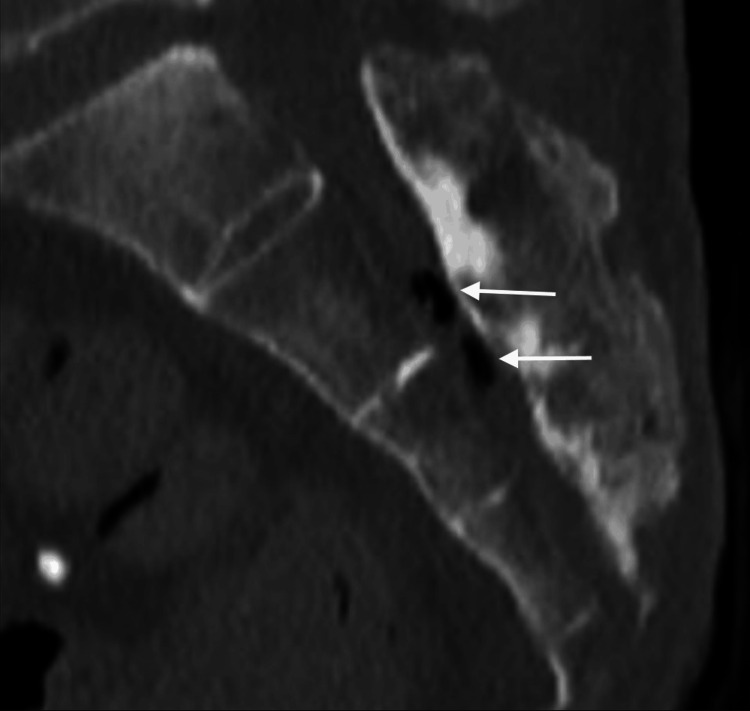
CT scan of sacrum and coccyx at different sagittal plane Osteomyelitis of distal sacrum and coccyx with gas formation in spinal canal visualized on CT at a different sagittal plane level with gas ascending the spinal canal suggesting ascending spinal canal infection (white arrows).
